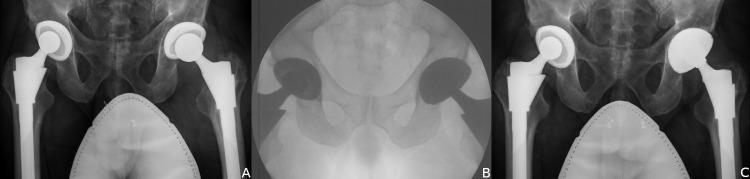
Management of left hip recurrent dislocation. (A) AP radiograph in a patient with recurrent dislocation despite prior revision with a modular head exchange. (B) Intraoperative fluoroscopy confirming cup orientation, offset and leg length. (C) AP radiograph three years after MDM revision with no further dislocations.
